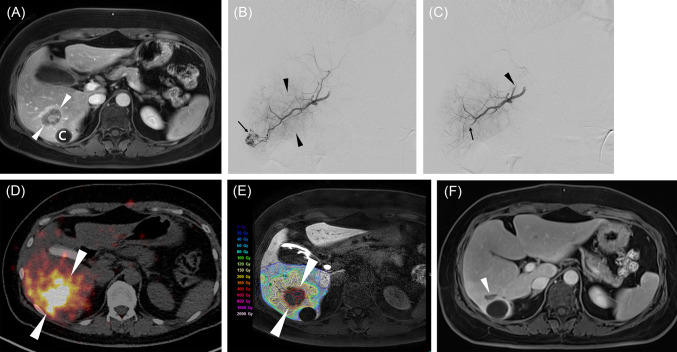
A 51-year-old woman with metastatic nodule from colon cancer. A Axial MR image of portal phase shows a 3.5 cm tumor in segment VI (arrowheads). Note simple hepatic cyst (C). B Selective angiogram of right posterior sectional artery shows tumor blush (arrowheads), and hemangioma (arrow). Segment VII artery and hemangioma was embolized with gelatin sponge particles. C Resin microspheres of 0.73 GBq were infused after embolization of segment VII artery (arrowhead) and hemangioma (arrow). D Y-90 PET/CT image obtained the next morning shows hot activity in the tumor of segment VI (arrowheads). E Dosimetric analysis image by Simplicity90 program shows hot activity in the tumor of segment VI (arrowheads). F Axial MR image of portal venous phase 6 months after radioembolization shows partial response of tumor in segment VI (arrowhead)
